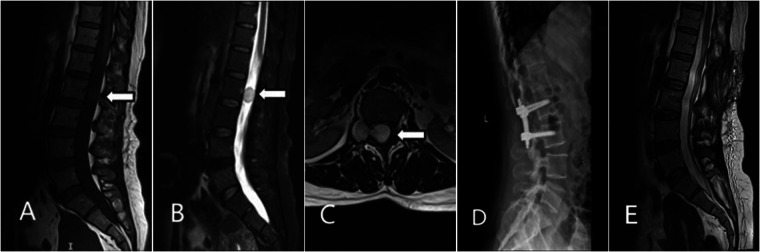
Imaging findings of the dumbbell-shaped tumor at L2–L3. (A) Sagittal T1WI showing the intraspinal tumor compressing the thecal sac; (B) Sagittal contrast-enhanced T2WI showing heterogeneous enhancement of the tumor; (C) Axial T2-weighted image at the L2–L3 level shows the tumor spanning both the intraspinal and extraspinal compartments, demonstrating a dumbbell morphology.; (D) Postoperative lateral radiograph demonstrates stable internal fixation at the L2–L3 level.; (E) Sagittal T2WI image at 1-year follow-up, showing no signs of recurrence.
